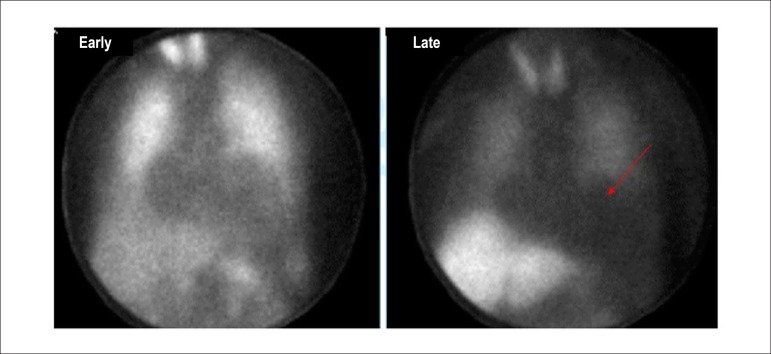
Abnormal cardiac scintigraphy with MIBG-^123^I of a patient with advanced heart failure and highly reduced radiopharmaceutical uptake in the cardiac area (arrow), which denotes cardiac sympathetic hyperactivity. Black and white images of the anterior thorax, acquired approximately 15 minutes (early) and 4 hours (late) following intravenous injection of the radiopharmaceutical MIBG-^123^I.
